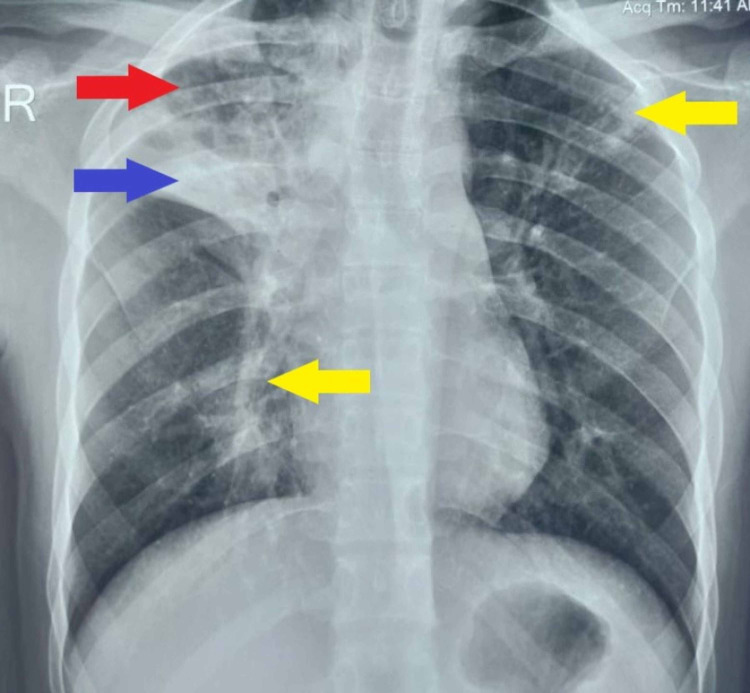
Radiograph of the chest showing a cavity (red arrow) and opacity (blue arrow) in the right upper zone along with bilateral areas of haziness (yellow arrows).
